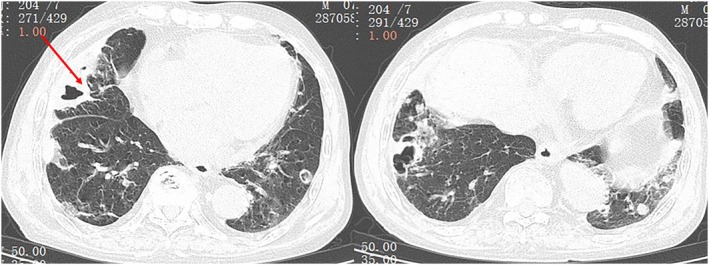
Thoracic computed tomography: Multiple peripheral or pleural based nodules in both lungs, ranging from a few millimetres to several centimetres, some with uneven‐walled cavities. A percutaneous needle biopsy was conducted on the arrow‐indicated lesion.
